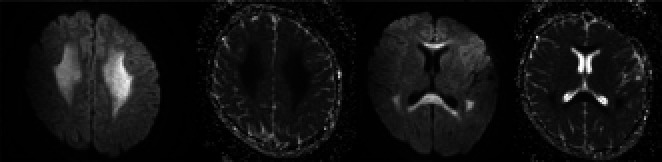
FIGURE 1 Initial brain MRI demonstrating DWI hyperintense and ADC hypointense lesions in the splenium of the corpus callosum and bilateral frontoparietal deep white matter.
